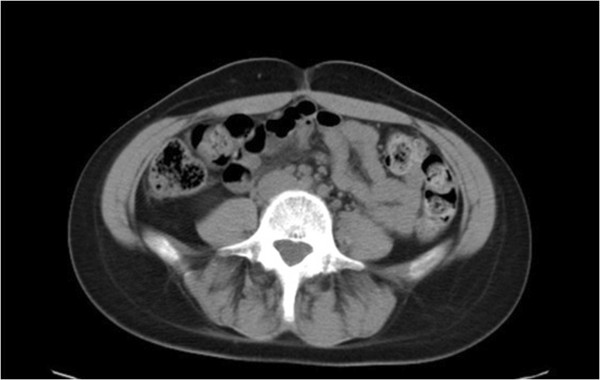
Abdominopelvic CT scan where the complete resolution of the haematoma can be observed.
